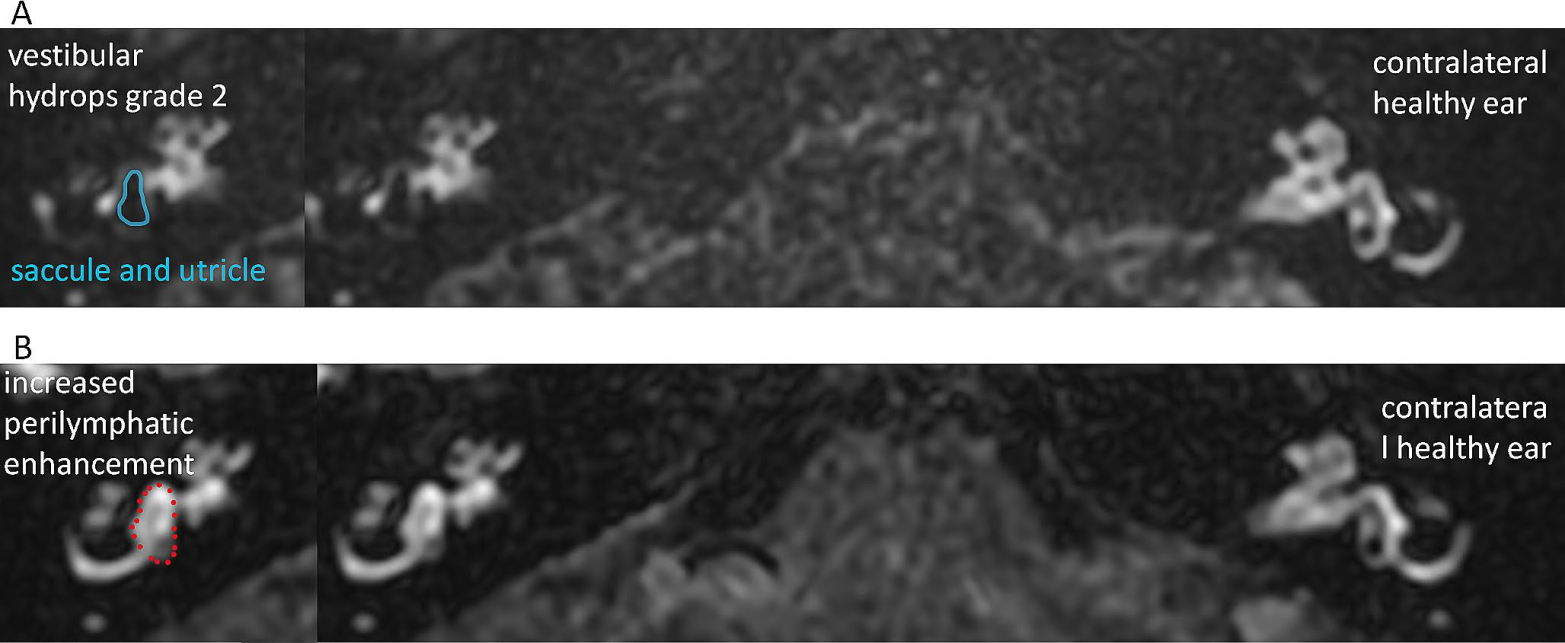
Magnetic resonance imaging (MRI) of a patient with a clinical diagnosis of definite Ménière’s disease in the right ear (case #14) before and 2.16 years after vestibular neurectomy (axial plane, delayed Gd-enhanced images). Panel (A) Preoperative images showing dilated saccule confluent with utricle with circular enhancing perilymphatic space – vestibular endolymphatic hydrops grade 2 according to Bernaerts scale; Panel (B) A severe asymmetrical perilymphatic enhancement in the vestibule preventing a reliable vestibular endolymphatic space evaluation in the postoperative examination. On both panels, the small left side images show the same images of the right analyzed ears, respectively, in the form of companion scans with some line art applied to define specific anatomical structures for easier identification of observed pathologies
